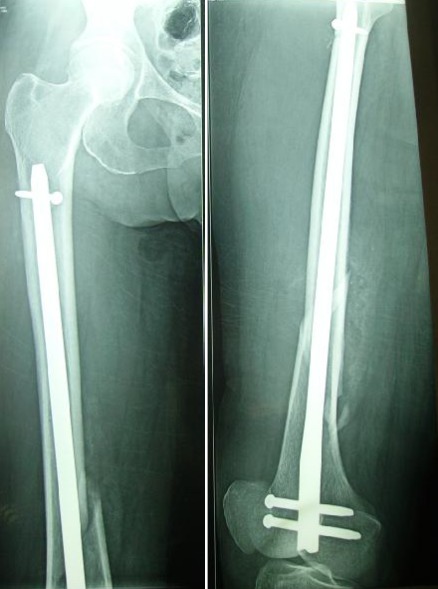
Radiographie postopératoire montrant l’ostéosynthèse par un clou rétrograde avec double verrouillage distal et proximal
